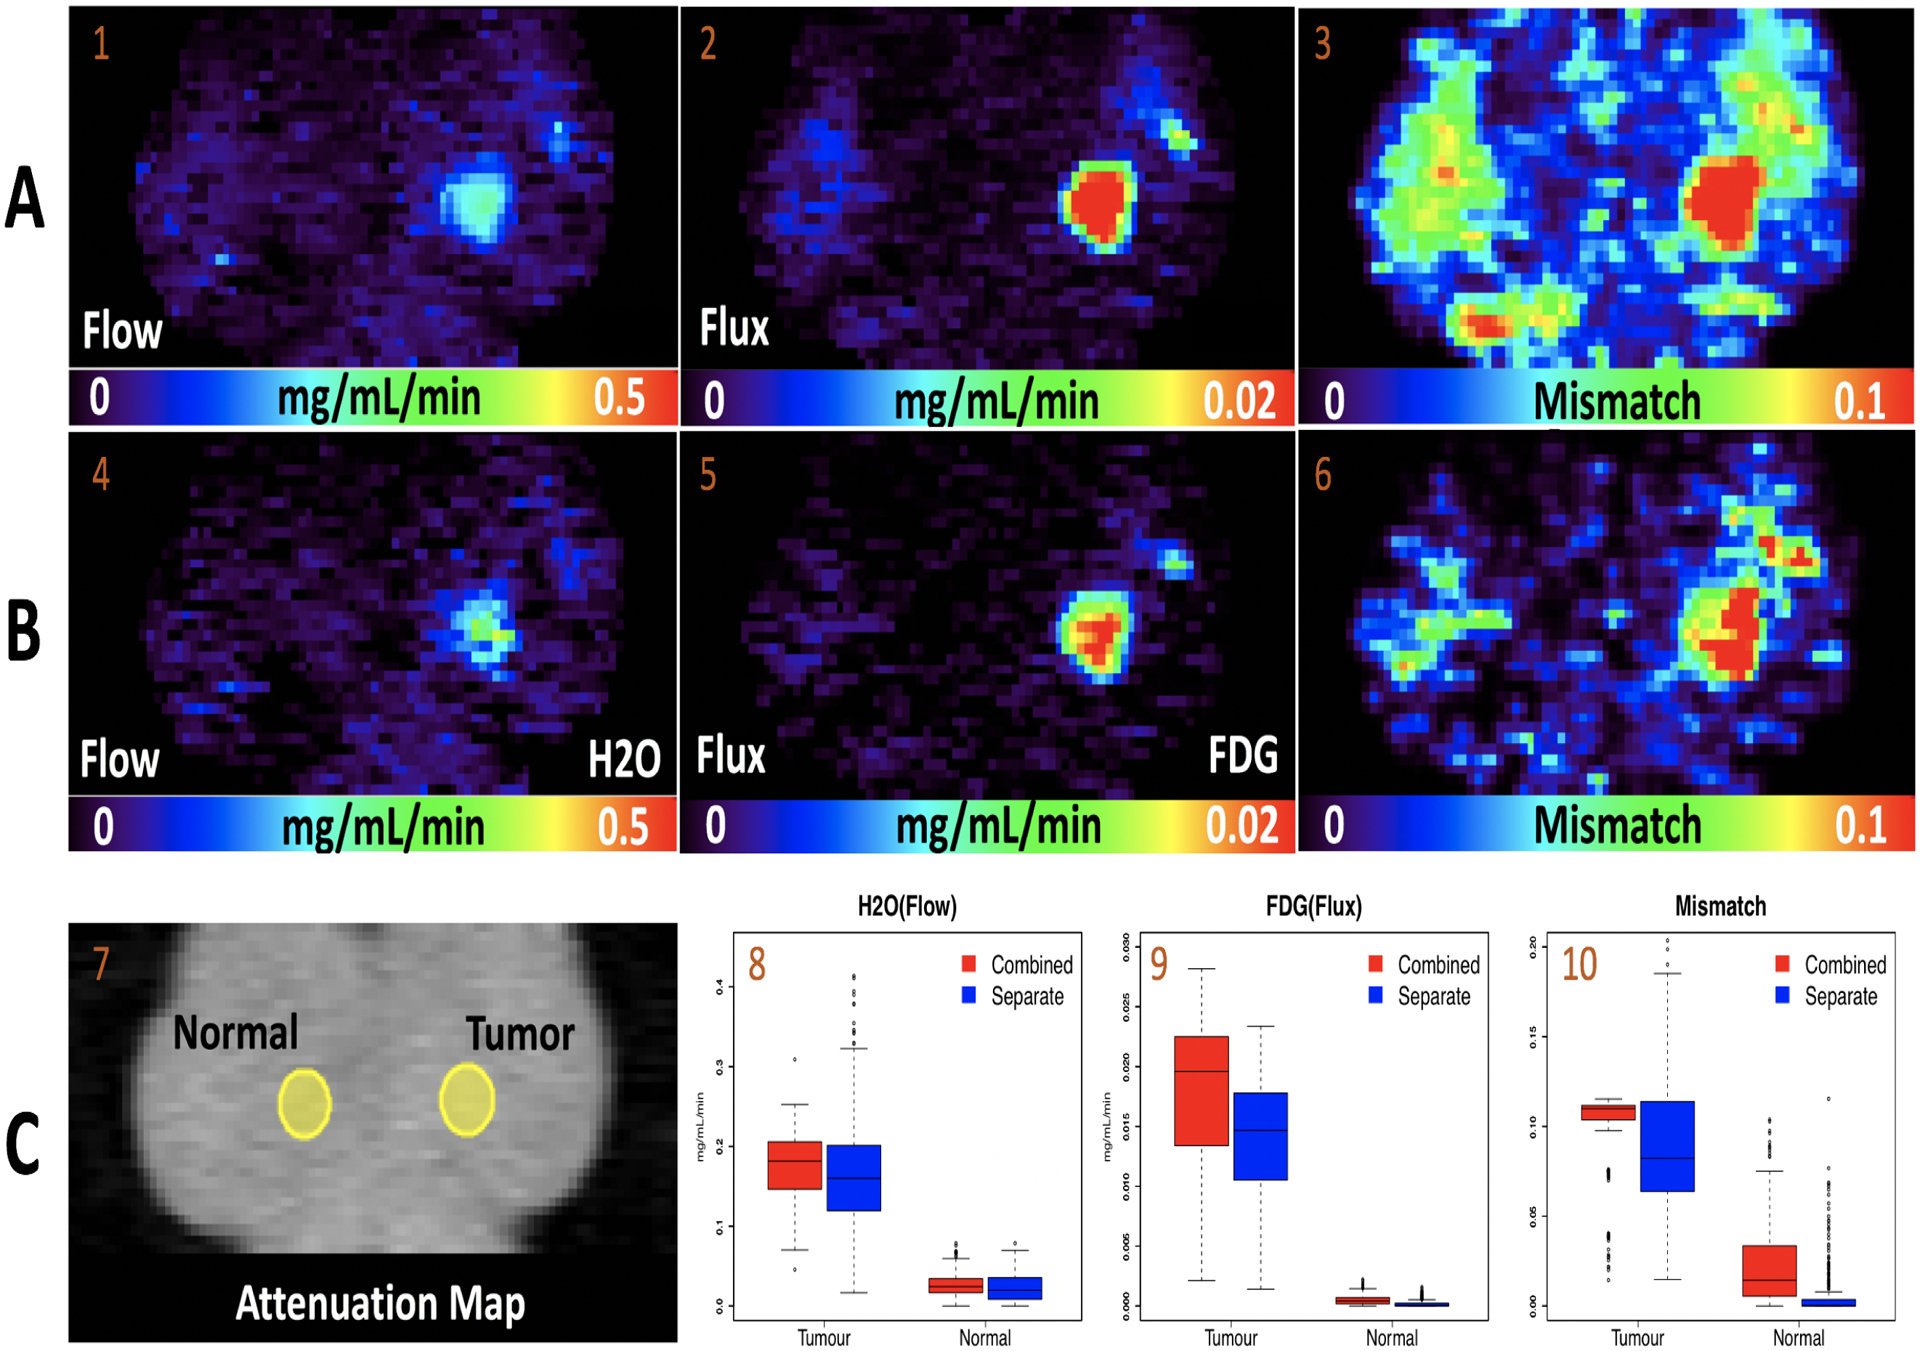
Estimates of flow, flux and mismatch in a H_2_O-FDG breast cancer study from combined and separate analysis. Row A: Combined Analysis; Row B: Separate Analysis; Row C: ROI Analysis.
